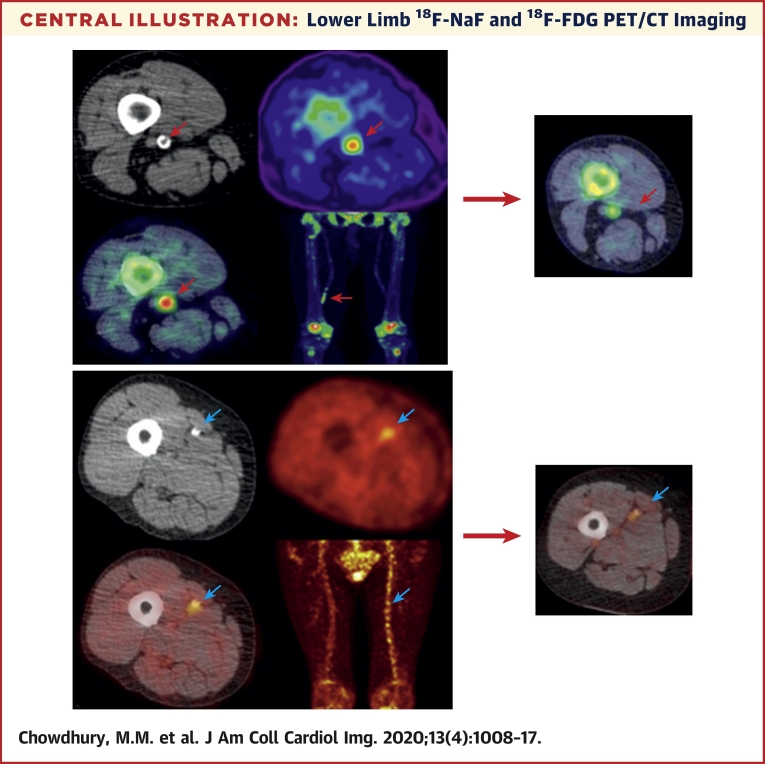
Lower Limb ^18^F-NaF and ^18^F-FDG PET/CT Imaging Non-contrast CT (top left) with a rim of calcification of the vessel, ^18^F-NaF PET (top right), and fused ^18^F-NaF PET/CT (bottom left) of the superficial femoral artery (red arrow) at the level of the adductor canal, demonstrating significant vessel uptake in patient with claudication at 300 yards. In addition, there is prominent uptake seen in the vessel at the same level on the coronal image (bottom right). The same plaque imaged at 6 weeks post angioplasty demonstrates dampened signal uptake (far right, red arrow), with a patient who did not develop restenosis. Non-contrast CT (top left) with calcification of the vessel, ^18^F-FDG PET (top right) and fused ^18^F-NaF PET/CT (bottom left) of the superficial femoral artery (blue arrow) at the level mid-thigh, demonstrating significant vessel uptake in a patient with tissue loss in the left leg. In addition, there is prominent uptake seen across the whole vessel coronal image (bottom right), in comparison with the contralateral leg. The same plaque imaged at 6 weeks post angioplasty demonstrates a persistent signal uptake (far right), in a patient who did develop restenosis. CT = computed tomography; ^18^F-FDG = ^18^F-fluorodeoxyglucose; ^18^F-NaF = ^18^F-sodium fluoride; PET = positron emission tomography.
